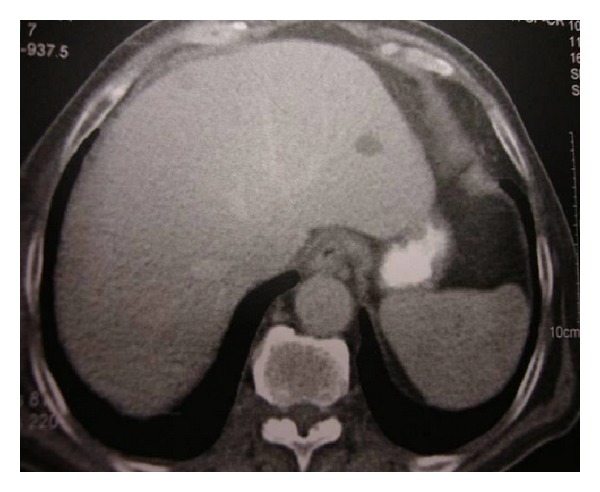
Abdominal CT imaging 3 months after administration of anti-TB agents, showing reduction of the hypodense lesions and of the retroperitoneal lymphadenopathy.
